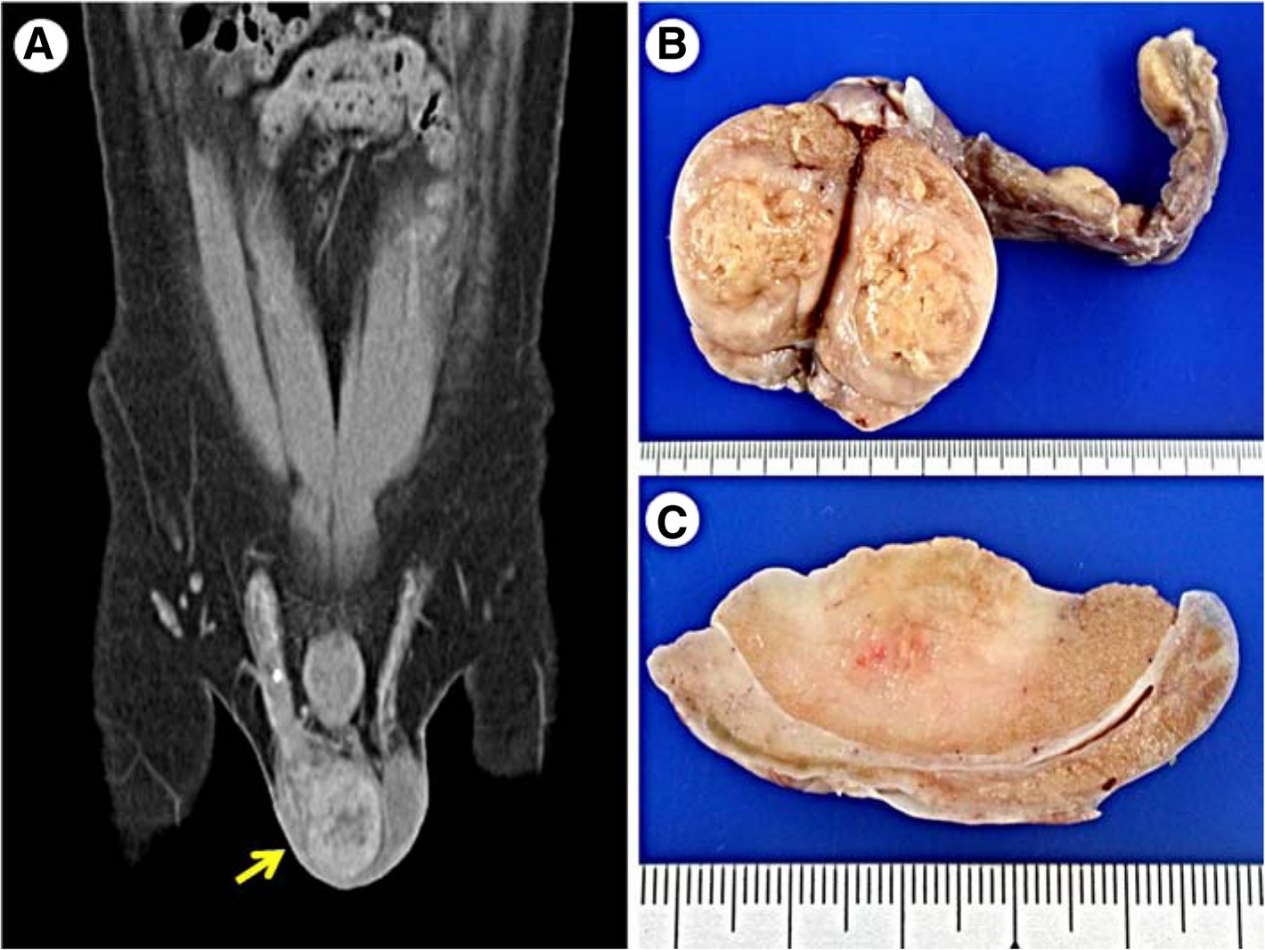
a Contrast CT shows enlargement of right testis with heterogeneous enhancement (*arrow*). b The testis shows a gray and yellow bulging tumor on cut surface. c The border of the tumor is infiltrative
